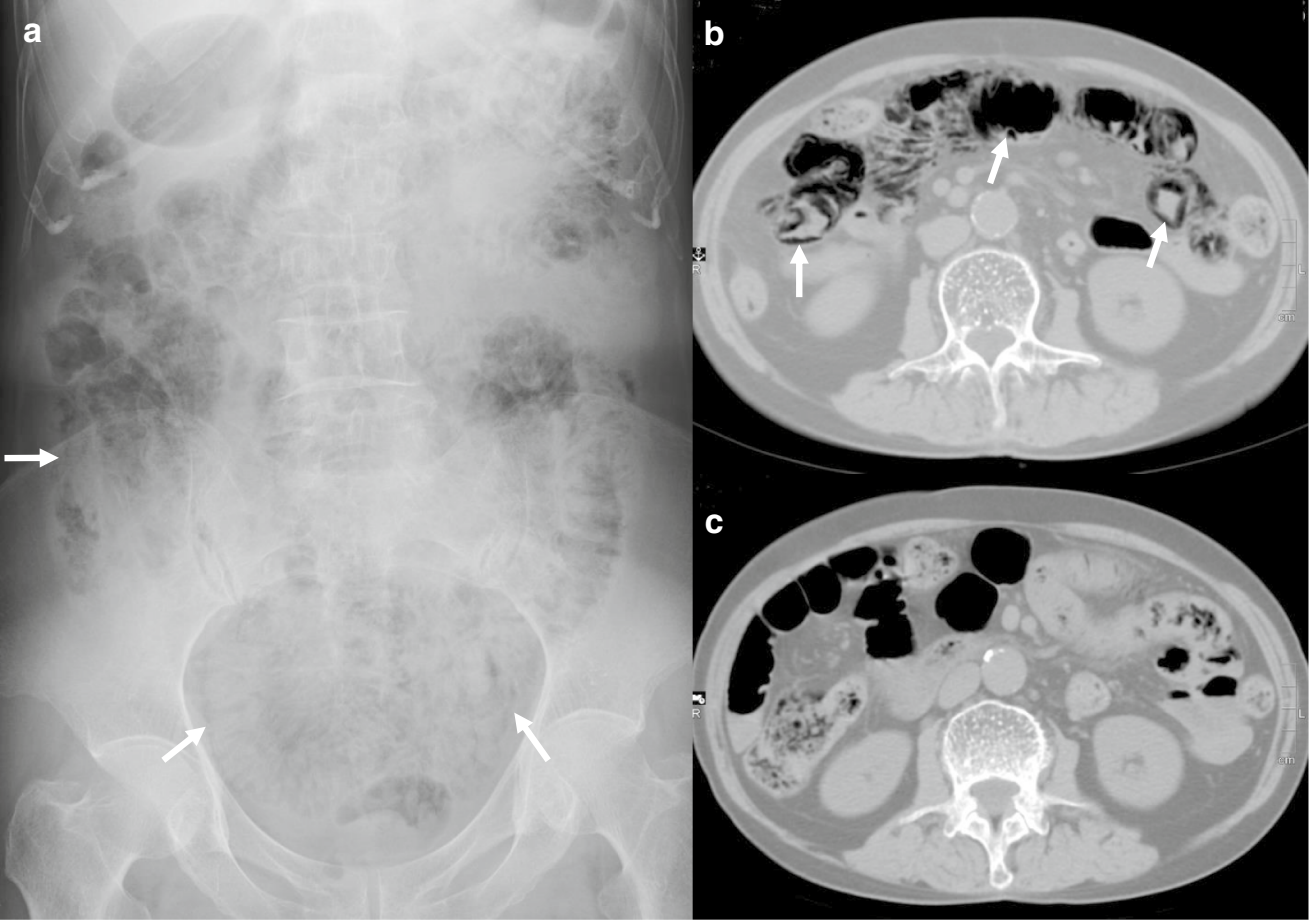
Plain abdominal radiographic and abdominal computed tomography (CT) images of case 2. a Plain abdominal radiograph showed several radiolucent tracks along the wall of the small intestine (*white arrows*). b CT of the abdomen under a wide window setting showed intramural gas widely distributed in the small intestine (*white arrows*). c Intramural gas in the small intestine almost disappeared after conservative therapy for 11 days
